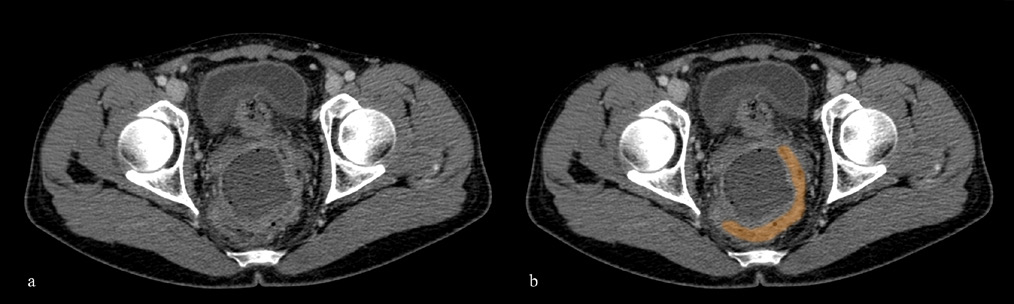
(a) Axial post-contrast CT scan in portal venous phase shows the sigmarectum (highlighted area in b) compressed and dislocated by the abscess in left iliac fossa.
